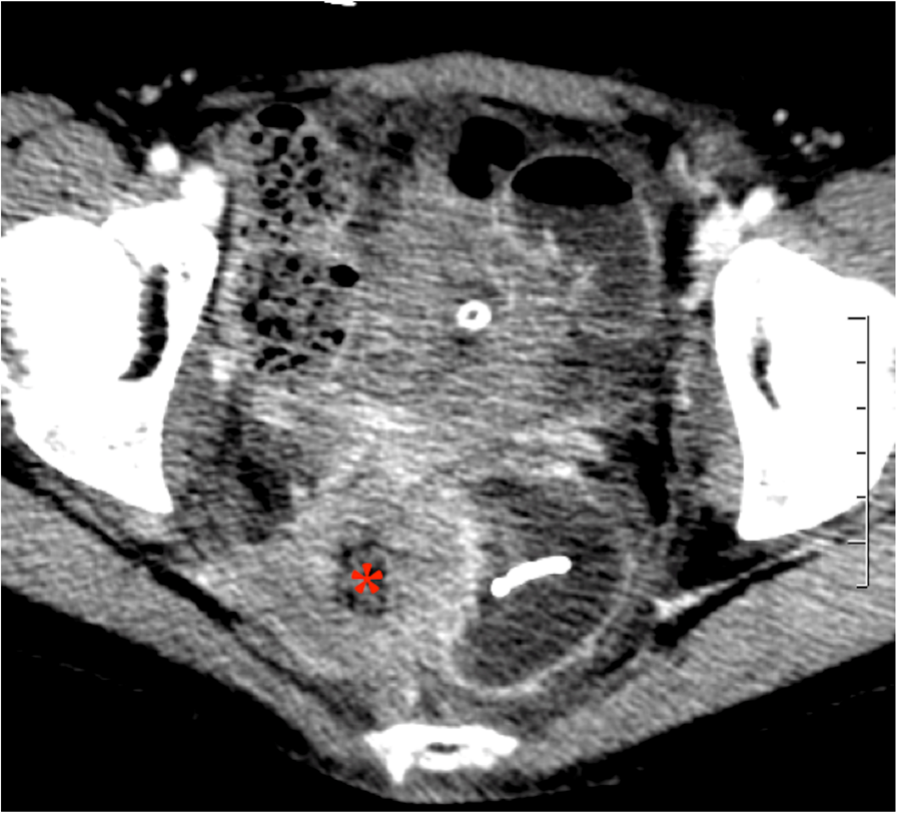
Axial section of the CT scan in a venous phase demonstrating a right sided pelvic tumor recurrence with a partial central necrosis. The distal coil of the stent inside the rectal lumen and the bladder catheter are also visible
